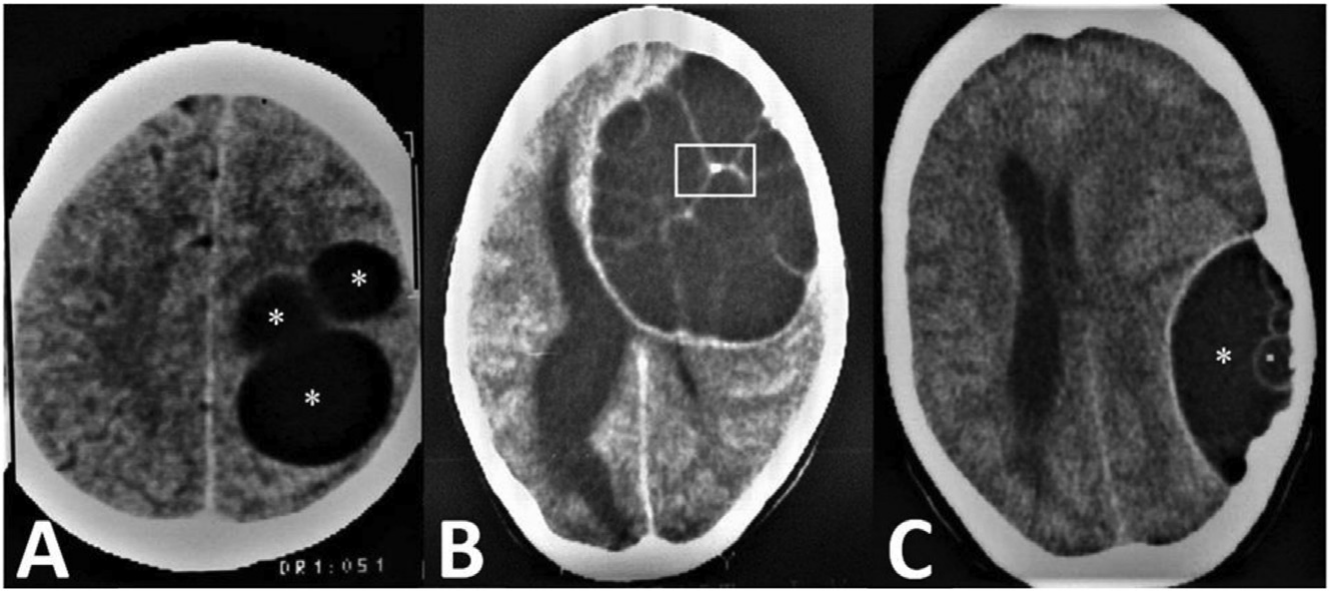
Axial brain CT scans shows A) multiple hydatid cysts (asterisks) in a 45-year-old woman, B) a multiloculated hydatid cyst with septal calcification (rectangle) in a 12-y/o girl, C) post-surgical recurrence of hydatid cyst presenting as a multiloculated extra-axial lesion (Asterisk) in a 40-y/o woman. (Photos are from our own patient(s) and they had not been published previously elsewhere.).
